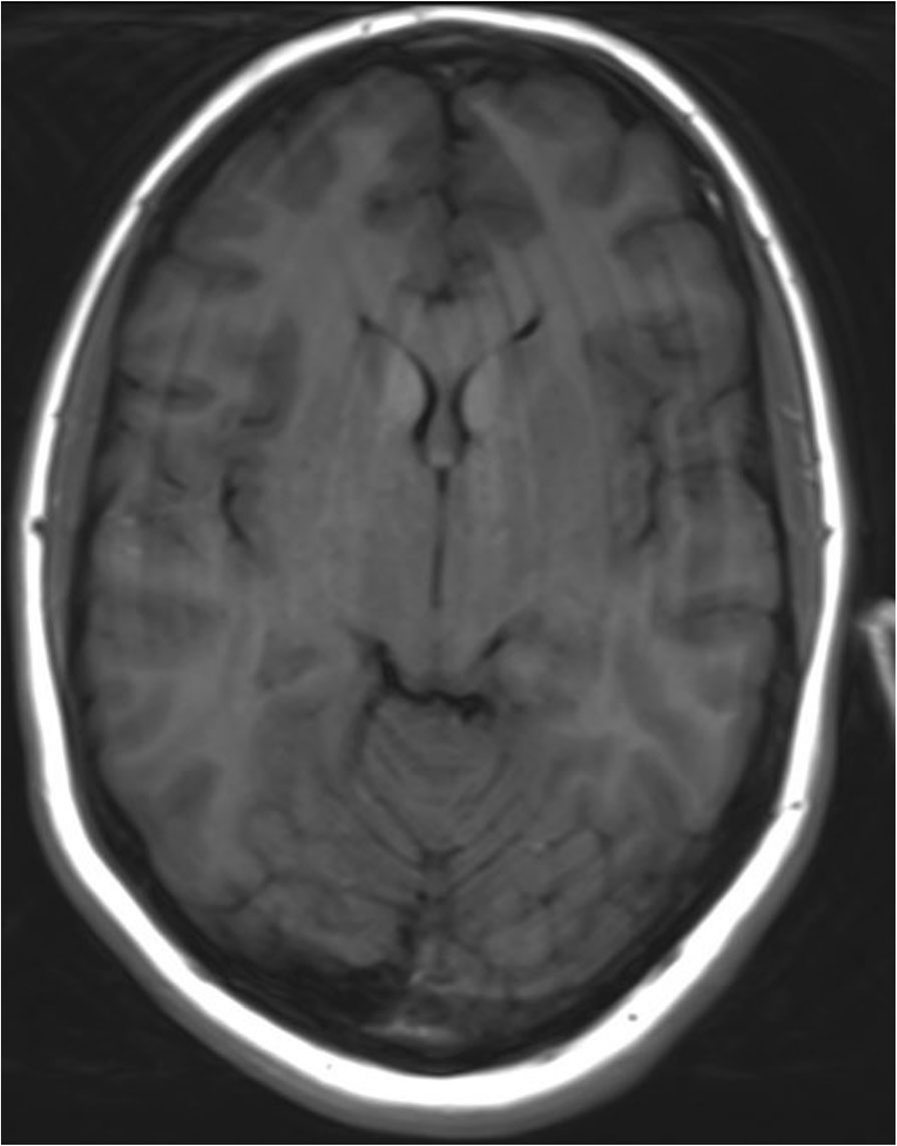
Corresponding MR T1 sequences show hyperintense calcifications in the same regions as those seen on CT examination
